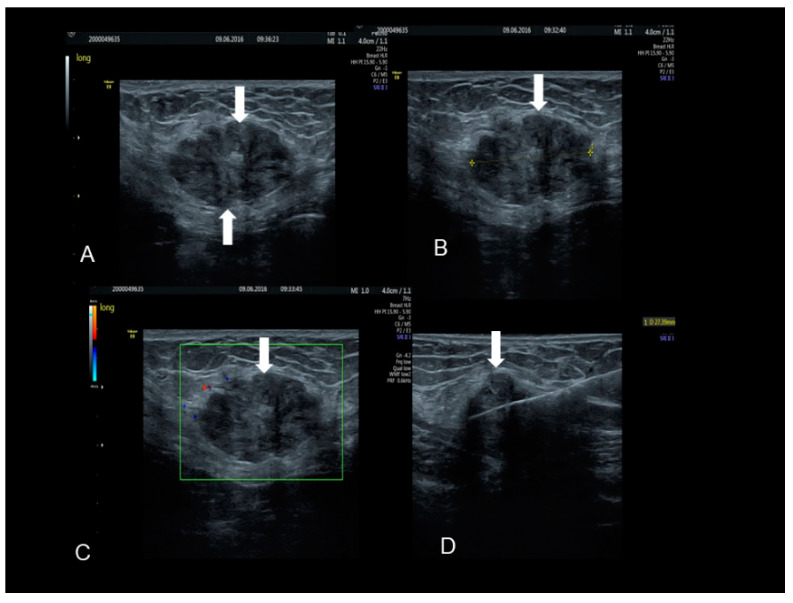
The ultrasonographic appearance of a rectus abdominis endometriotic nodule (see white arrows) (A–D) in a woman with previous cesarean section and hysterectomy. The last picture (D) is taken during the needle biopsy.
